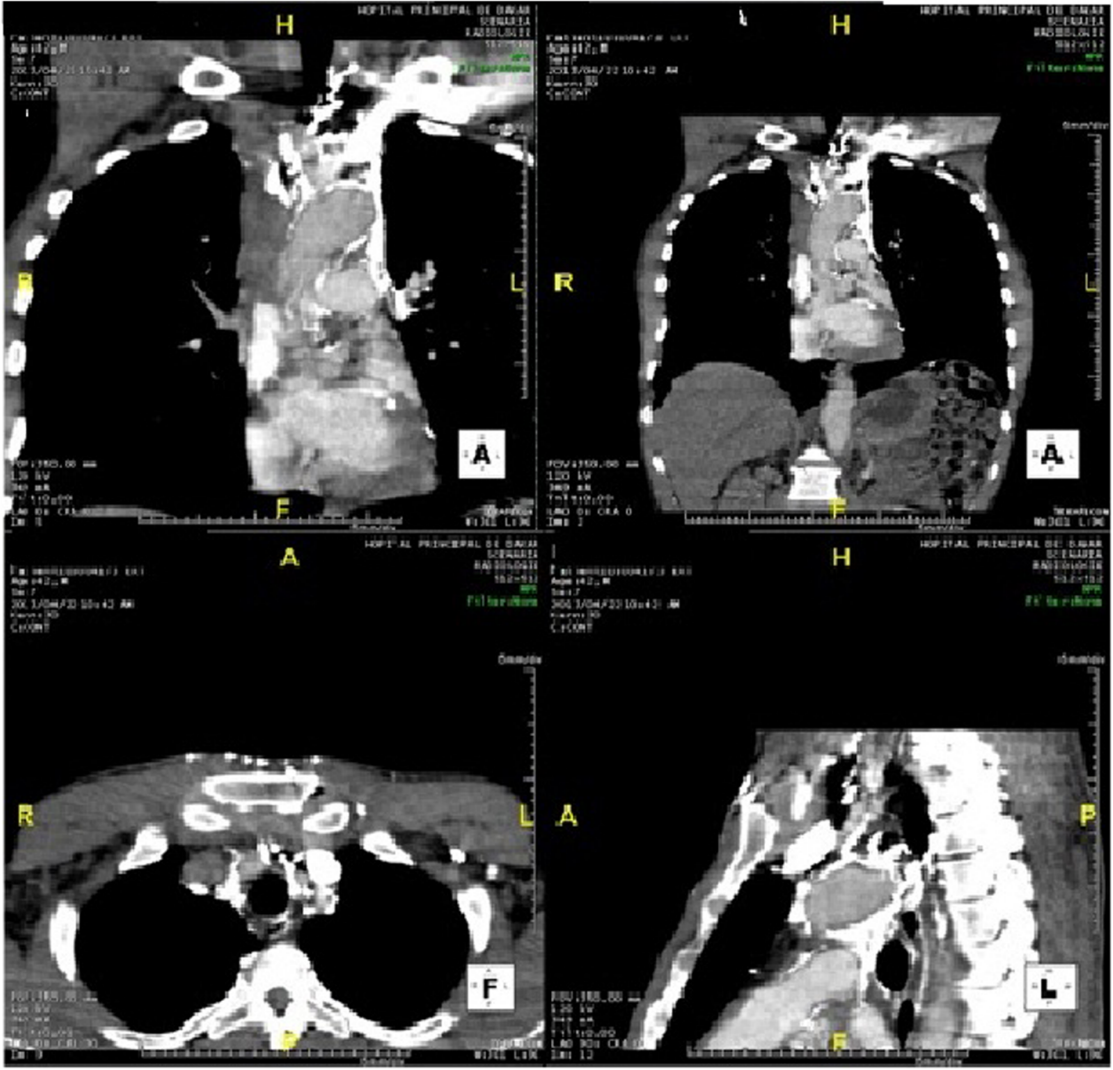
Thoracic CT angiography showing an internal jugular and superior vena thrombosis.
